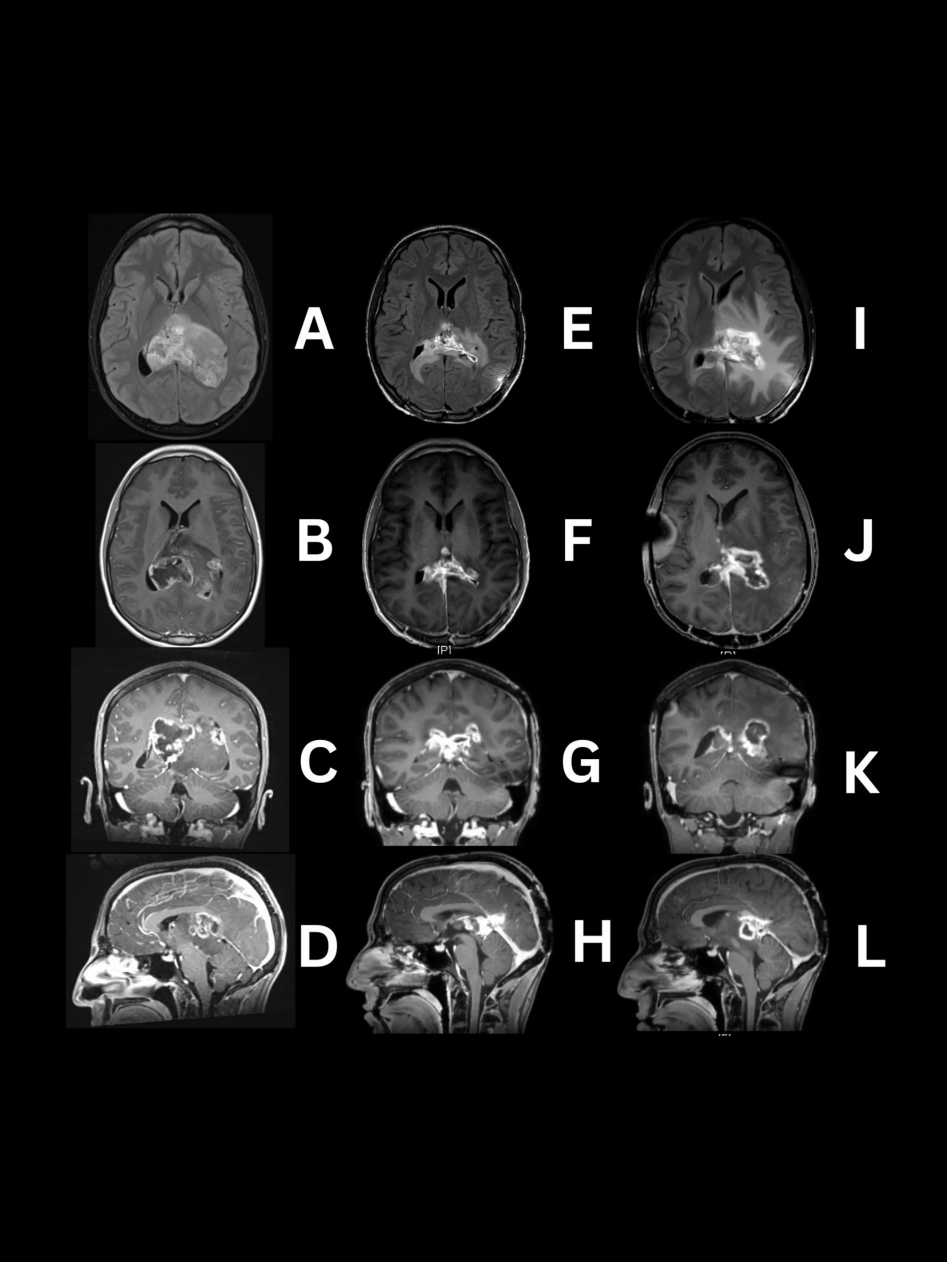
MRI images demonstrating serial progression of H3 K27-altered diffuse midline glioma. Preoperative (A–D) tumor located in lateral ventricles predominantly solid with patchy areas showing high intensity and central necrosis on the right side on T2/FLAIR and T1W post contrast. Day 104 post operation and chemoradiotherapy (E–H) reduced intraventricular tumor with periventricular FLAIR signal abnormality and contrast enhancement extending to the surrounding structures in T1W post-contrast images. Day 199 post-final treatment, (I–L) Progression of FLAIR signal (which is most likely a mixture of tumor infiltration with edema and radiation change) extending to basal ganglia and parietal lobes, with enhancement and necrosis of the intraventricular tumor and adjacent structures.
